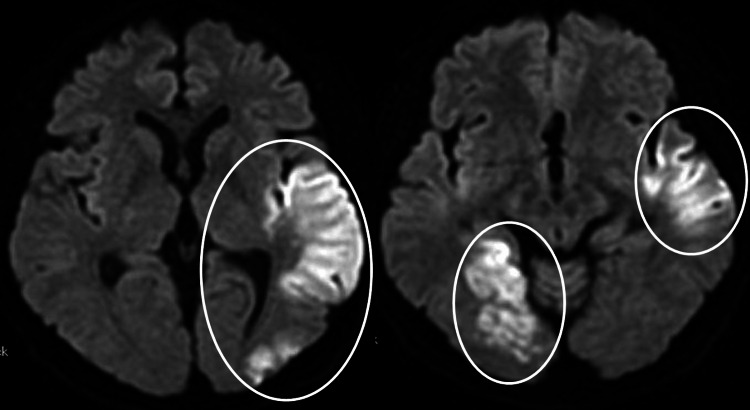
Brain MRI revealed multiple acute brain infarcts (circle).
